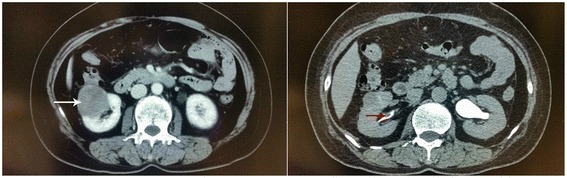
CT of a patient with concurrent renal cell carcinoma and ipsilateral pelvic carcinoma. The *white arrow* shows a renal mass in the right kidney; the *red arrow* shows a filling defect in the right renal pelvis
